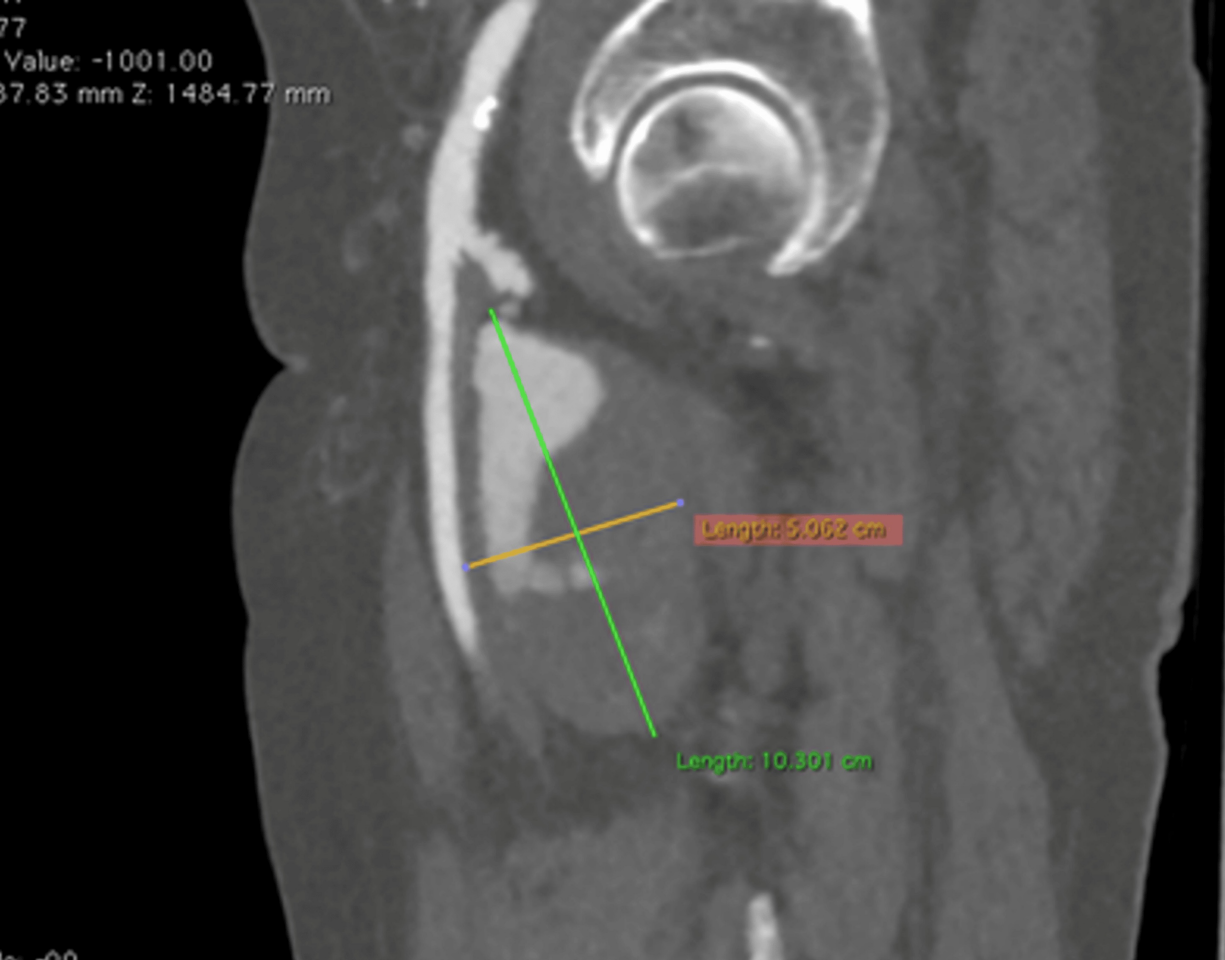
CT angiography, sagittal plane It shows the left deep femoral artery aneurysm with its craniocaudal and anteroposterior extension. Note the irregular mural thrombus, possibly due to multiple ulcerations.
